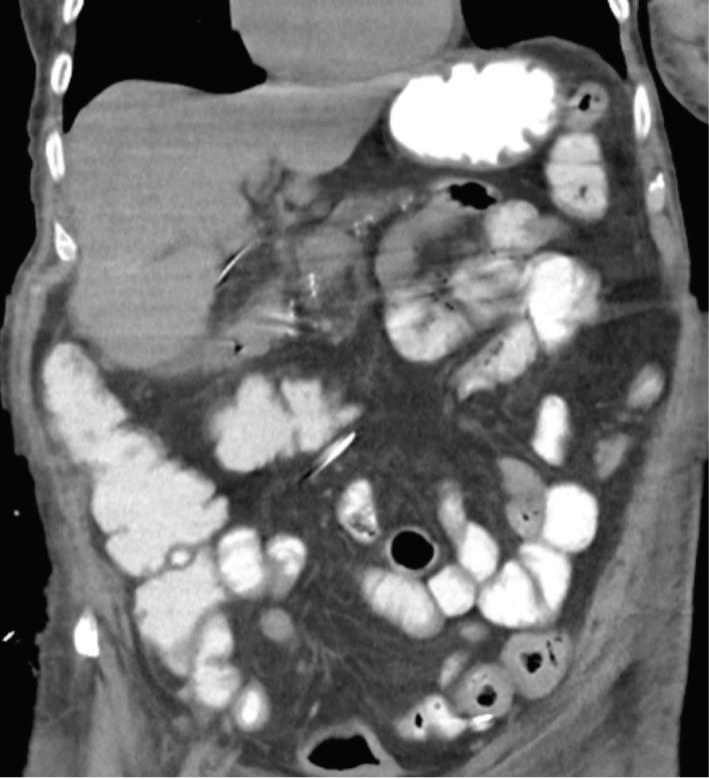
Oral contrast-enhanced CT on postoperative Day 7 demonstrating no contrast extravasation, indicative of the absence of an anastomotic or perforation-related leak.
